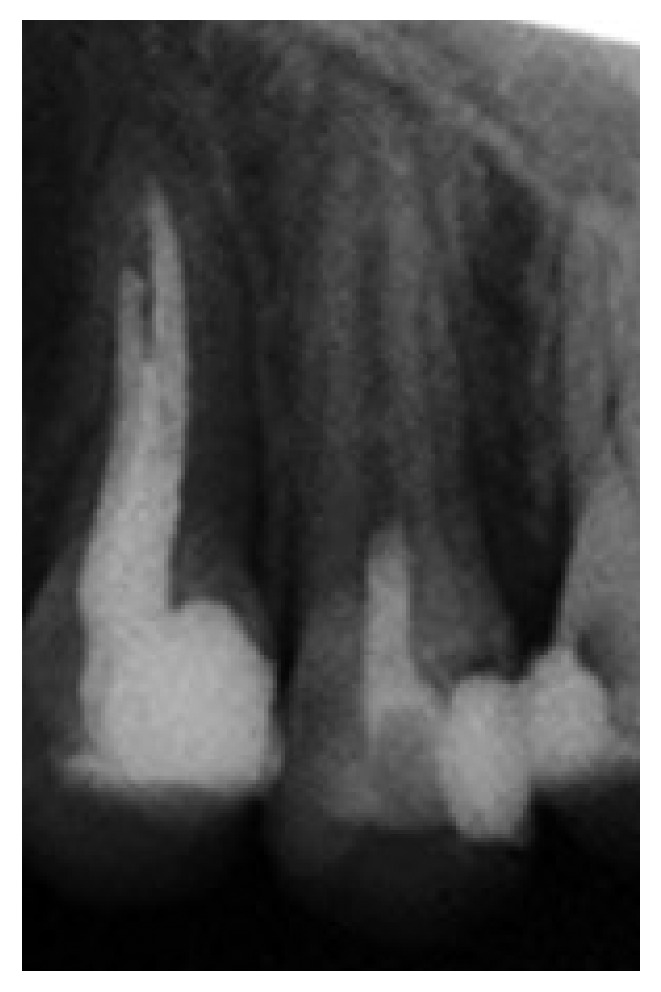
Six-month follow-up radiograph showing narrowing of the canal space with radiopaque shadows.
